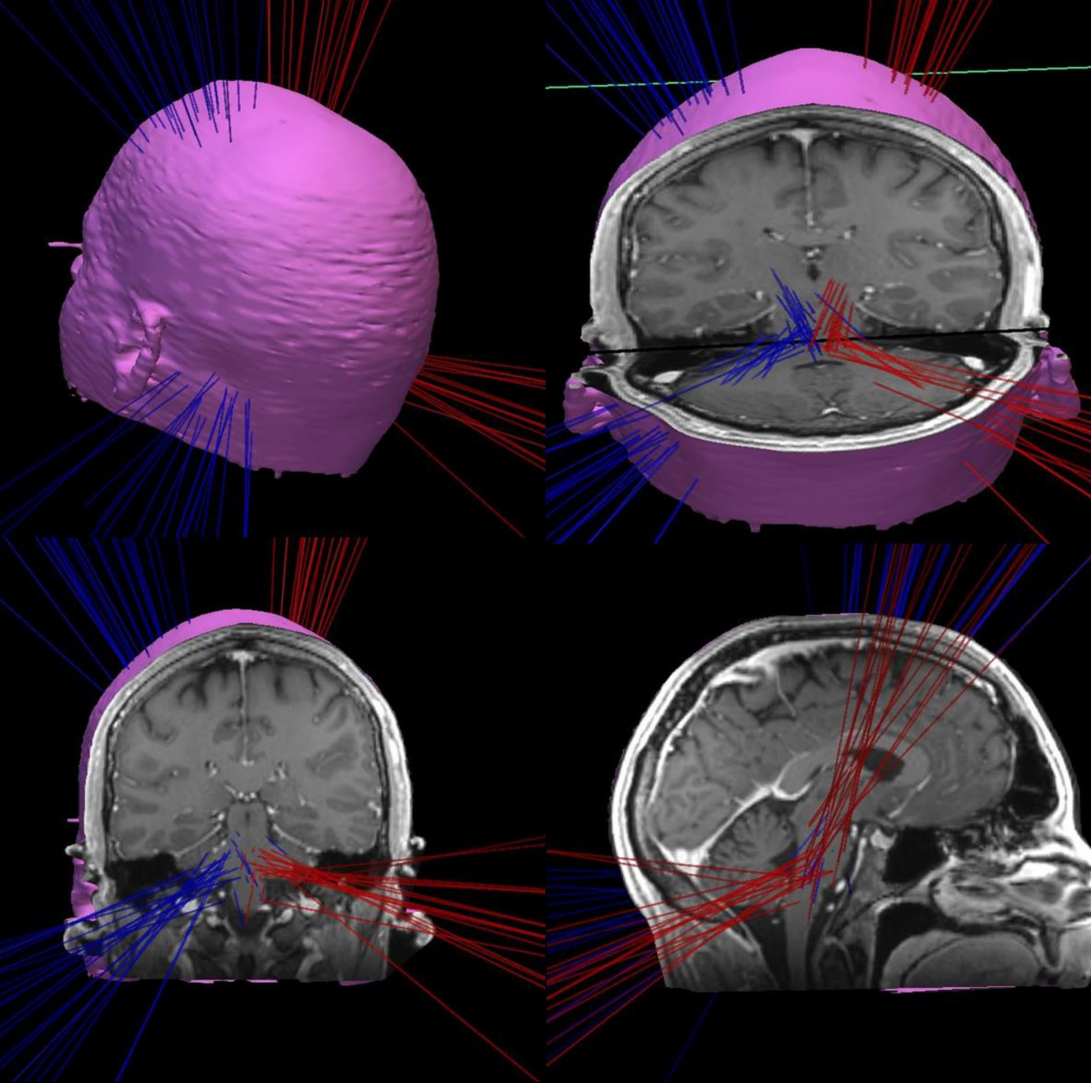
3D-model of the analyzed trajectories projected in a T1-MRI. Red = trajectories with entry point on the right side, blue = trajectories with entry point on the left side
